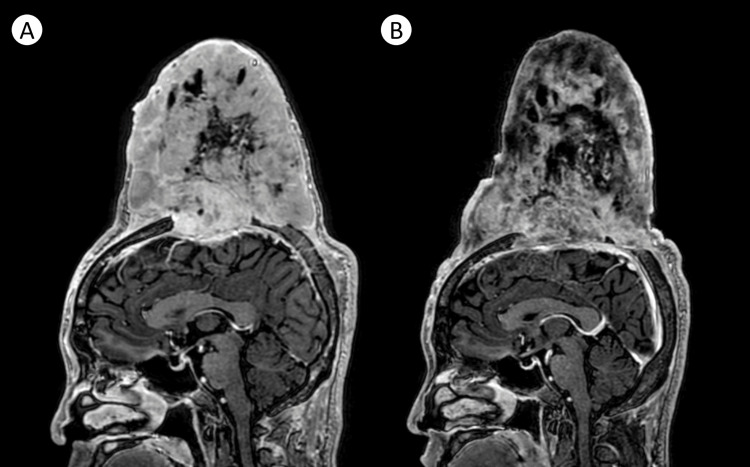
Representative sagittal T1-weighted MRI images of the tumor prior to treatment (A) and four months post-imatinib (B), showing an overall reduction in tumoral volume and size, now measuring 12.4x12.0x14.3 cm (APxTxCC), with decreased enhancement cystic degeneration, attributable to tumor necrosis.
